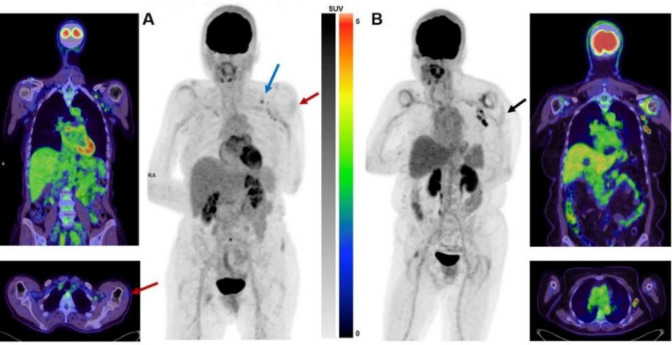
(A) A 62-year-old female with a new sacral nerve root enhancing lesion on MRI presents for further characterisation. There is a cluster of mild to moderately avid small left axillary lymph nodes at level1 (SUV_max_=3.1) and level 2 (SUV_max_=2.7) as well as left infraclavicular lymph node (SUV_max_=3.6) (Blue arrow). Mild activity is also seen in the superficial left deltoid muscle (SUV_max_=1.4) (Red arrows). The patient had Pfizer vaccination 6 days prior to PET scan injected to the left arm. (B) 67-year-old female with a poorly defined fat stranding in the right perinephric space suspicious for sarcoma presents for assessment. Multiple enlarged, intensely avid left axillary lymph nodes are noted (SUV_max_ up to 8.2). Low-grade activity is seen at the superficial aspect of the left deltoid (SUV_max_=1.5) (Black arrow). She had history of second dose Pfizer vaccination 5 days prior to PET scan
